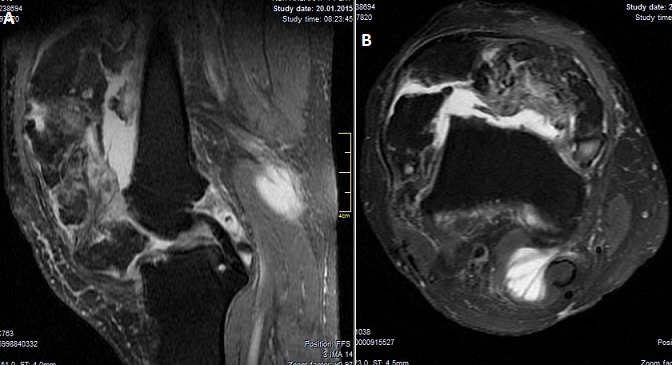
MRI T-2 image showing the effusion, synovial hypertrophy and a loose calcific body (a, b)
